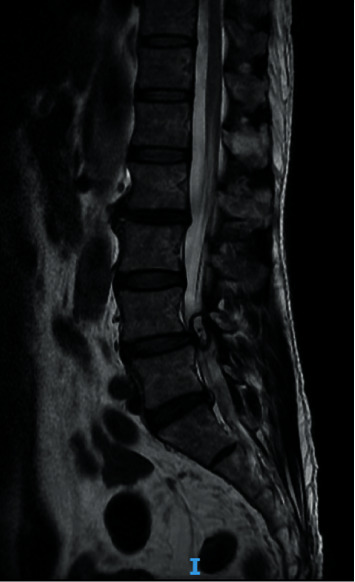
Preoperative magnetic resonance imaging (MRI) scan. This sagittal T2-weighted MRI image shows a hyperintense cystic lesion on the left side at the L4–5 level.
